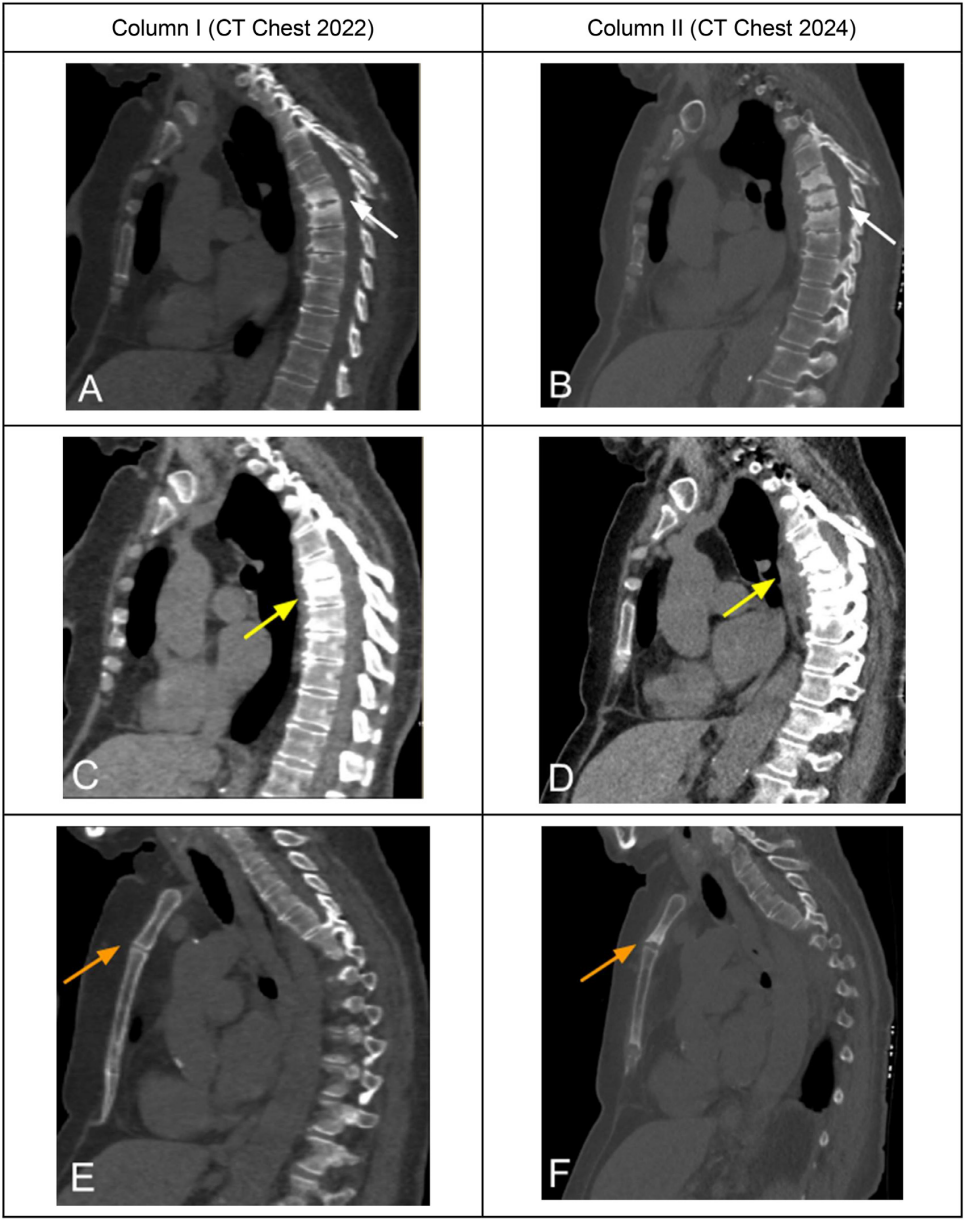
CT Chest 2022 in Column I and CT Chest 2024 in Column II showing progression of spread (A) CT Chest 2022 with bone window showing multilevel vertebral endplate sclerosis at T6-T7 (white arrows) (B) Shows interval increased vertebral sclerosis involving T5-T9 (white arrow). (C) Soft tissue window demonstrating thin paravertebral phlegmon (yellow arrow). (D) Interval increased paravertebral phlegmon (yellow arrow). (E) CT Chest 2022 has a lack of sternomanubrial findings (orange arrow) including sclerosis and soft tissue inflammation. (F) Shows interval development of sternomanubrial sclerosis (orange arrow) and soft tissue phlegmon (better visualized in Fig. 1).
